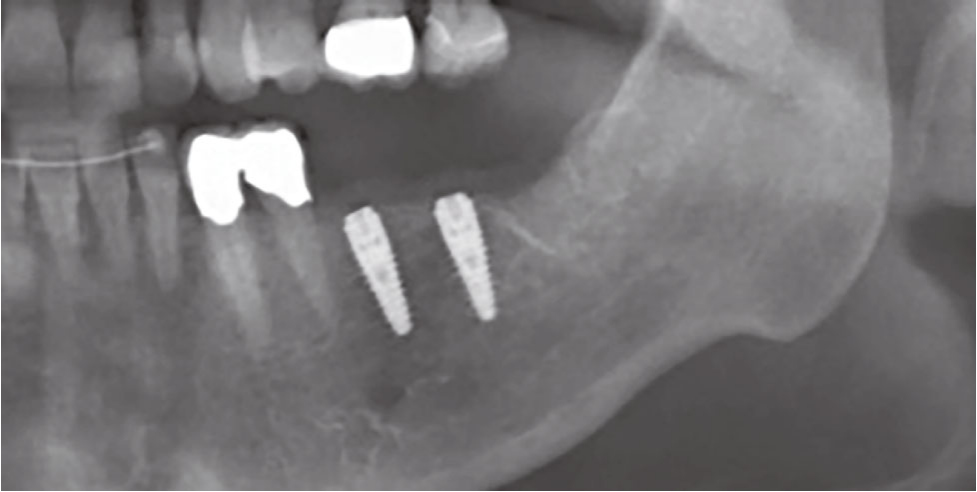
X-ray check after insertion.
